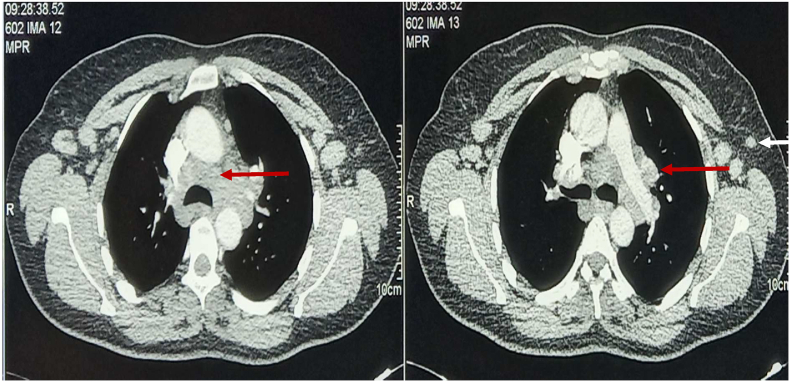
A thoracic CT scan showing non-compressive mediastinal and hilar adenopathy (red arrows) with axillary adenopathy (white arrow).
